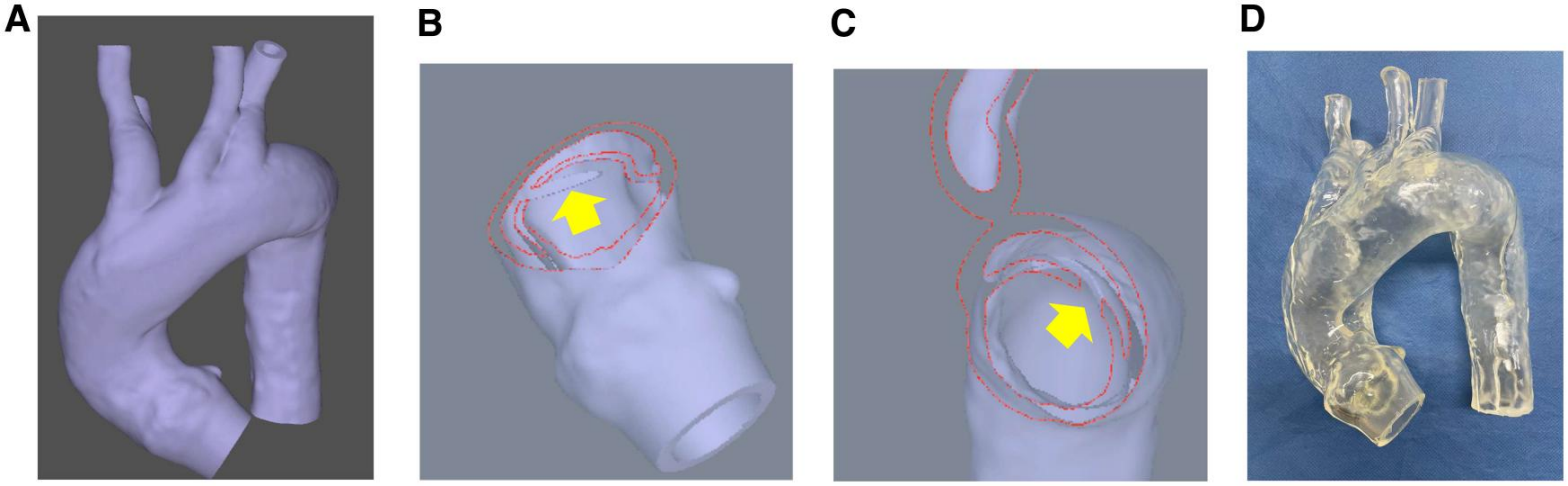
Manufacturing process of a three-dimensional (3D) polyurethane aortic dissection model. (A) Based on the 3D PDF from an actual patient’s images of computed tomography, the 3D soft model was manufactured. (B) An entry tear of 2 cm was located at the ascending aorta vertically in the type A aortic dissection model. (C) An entry tear of 2 cm was located at the descending aorta vertically. (D) The outer shapes of type A and B models are similar
